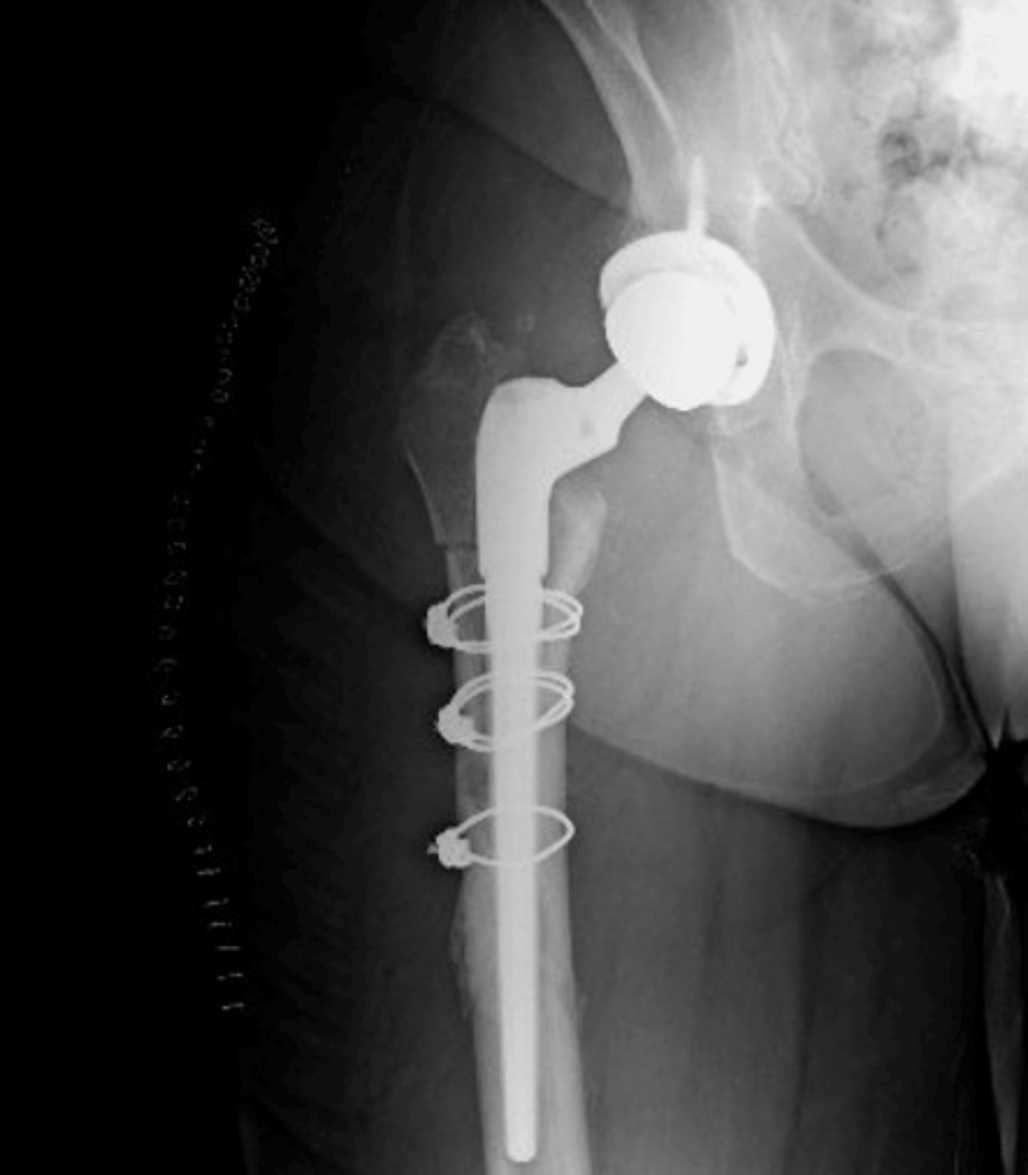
Postoperative anteroposterior X-ray of the right proximal femur demonstrating total hip revision arthroplasty result. A 36-mm cobalt-chrome femoral head, uncemented distal femoral stem 13 mm × 150 mm, and cone proximal body offset at 50 mm were used. To enhance stability, three cerclages of 1.8 mm × 914 mm were employed.
